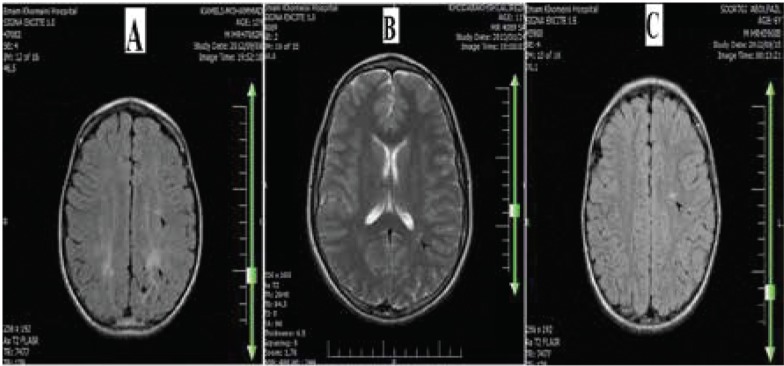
High signal areas in the periventricular white matter near the trigonearea of the lateral ventricles and semiovale centrum (A) periventricular white matter near the trigone area (B) semiovale centrum (C) T2 FLAIR MRI
